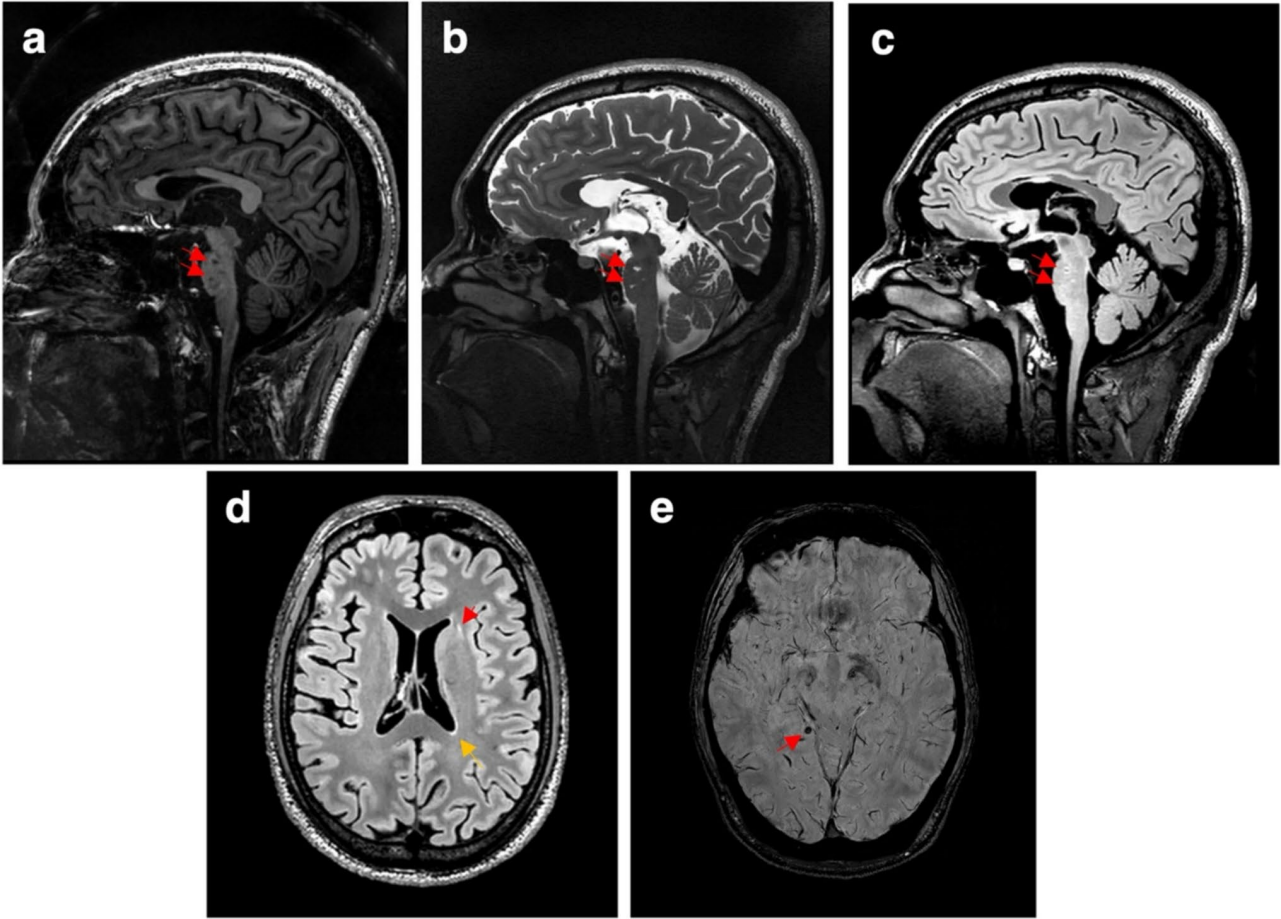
The CSVD imaging markers in DADA2 on 5.0 T MRI. a-c: The sagittal T1WI (a), T2WI (b), and FLAIR (c) show multiple lacunes (arrow) on the brainstem, and atrophy of the brainstem and cerebellum. d: The axial FLAIR shows dWMH (red arrow) and pWMH (yellow arrow). e: The SWI shows a CMB (arrow). All the imaging were from a 22-year-old male, 1 month after anti-TNF therapy. Abbreviations: CMB: cerebral microbleed, CSVD: cerebral small vessel disease, DADA2: Deficiency of adenosine deaminase 2, dWMH: deep white matter hyperintensities, FLAIR: fluid-attenuated inversion recovery imaging, MRI: magnetic resonance imaging, pWMH: periventricular white matter hyperintensities, T1WI: T1-weighted imaging, T2WI: T2-weighted imaging, SWI: sensitivity weighted images.
